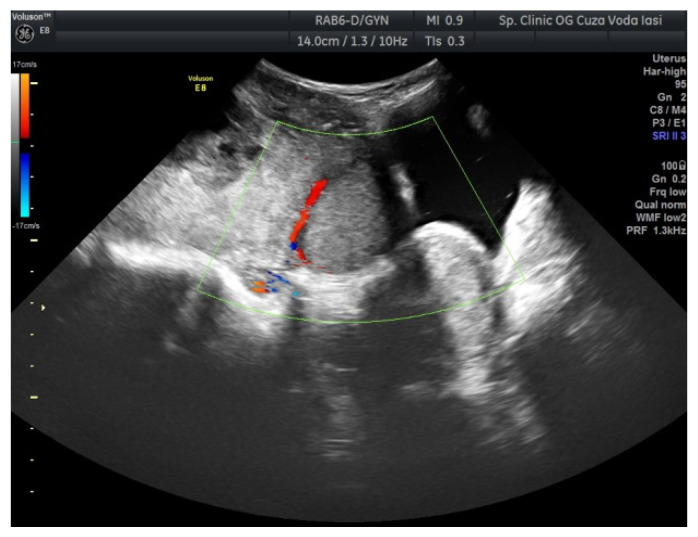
Ovarian color Doppler revealing blood vessels inside the solid part of the tumor (right ovary).
